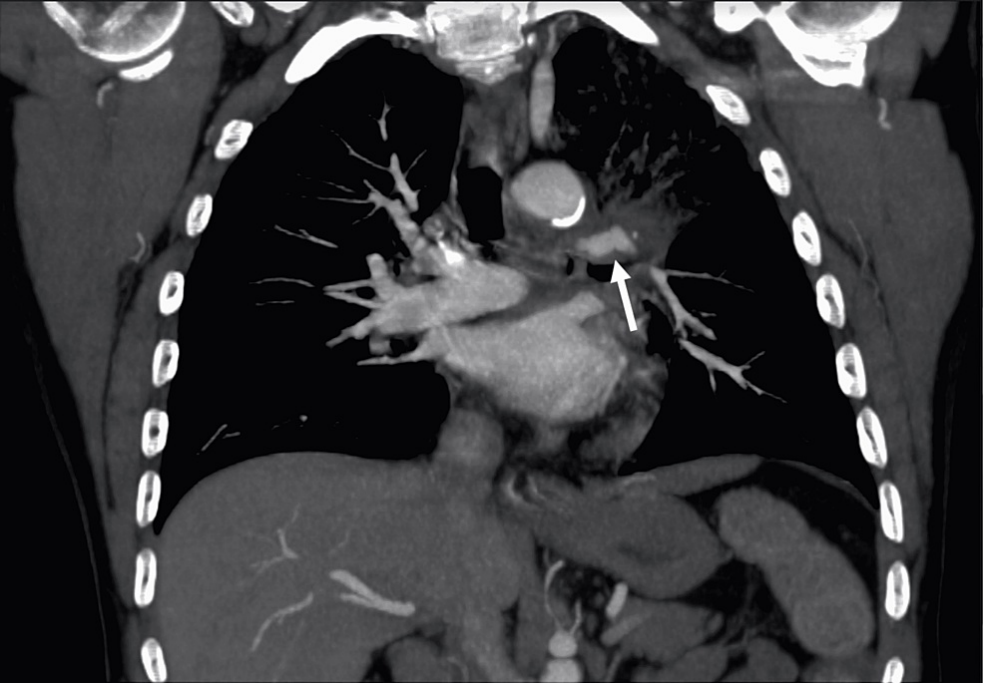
Coronal CT pulmonary angiogram showing the left suprahilar mass surrounding the left pulmonary artery with an associated pseudoaneurysm (arrow).
